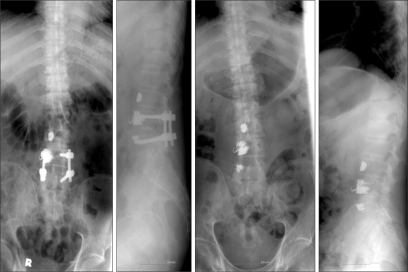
Preoperative and postoperative X-rays for the second operation. After posterior instruments were removed, we performed curettage and grafting with autologous iliac bone at L4-L5 and T10-T11.
